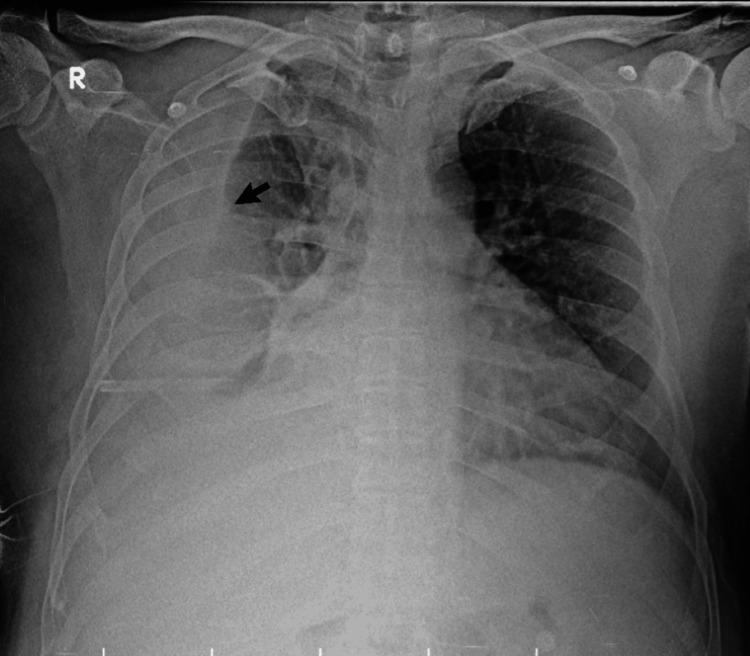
Initial chest radiograph. Anteroposterior chest radiograph obtained at presentation demonstrating a massive right-sided pleural effusion (arrow), presenting as a dense homogenous opacity that obliterates the right hemidiaphragm and costophrenic angle.
